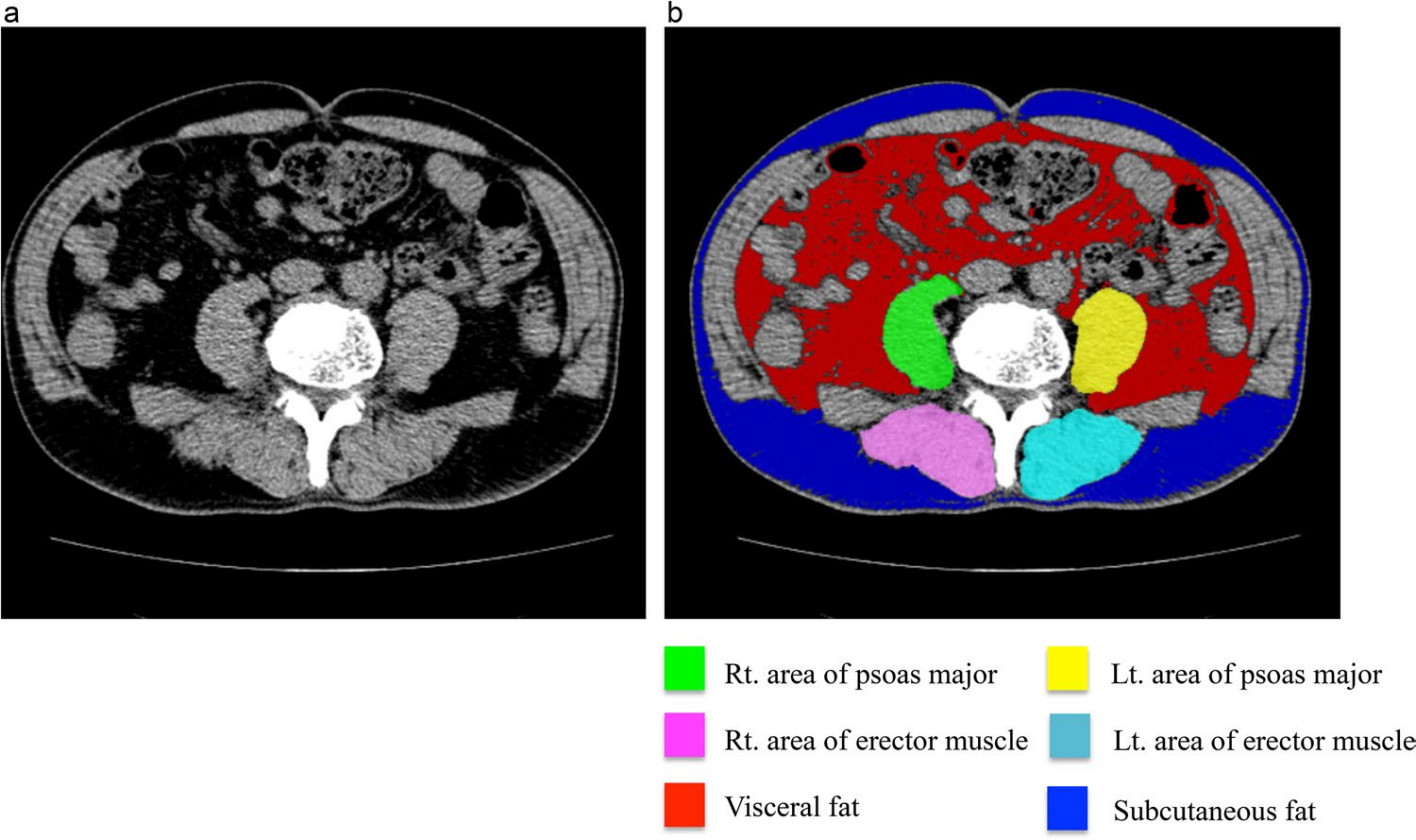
Representative view with musclePointer software. (a) An original image at the level of the umbilicus as observed using computed tomography. (b) Software calculated the area of each muscle automatically.
